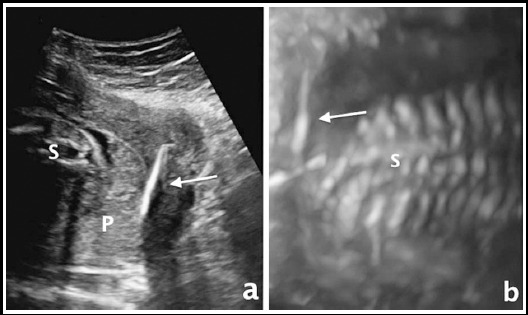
a) The embedded IUD between placenta and myometrium was shown via Gray Scale ultrasound. b) the closeness of IUD and spine of the fetus was shown by 3-dimentional ultrasound examinations with maximum (skeletal) mode. Both images were obtained from same patient who has 25 gestational weeks pregnancy with complicated by PPROM. Arrow: Intrauterine Device, S: Spine, P: Placenta, PPROM: Preterm premature rupture of membrane.
